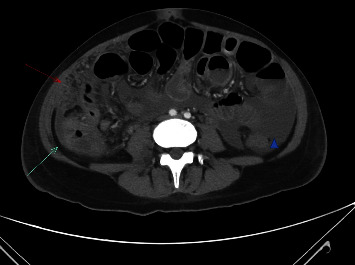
Abdominal findings associated with peritoneal tuberculosis on CT. Omental thickening (red arrow) and peritoneal thickening (green arrow) are noted along with ascites (blue arrowhead).
